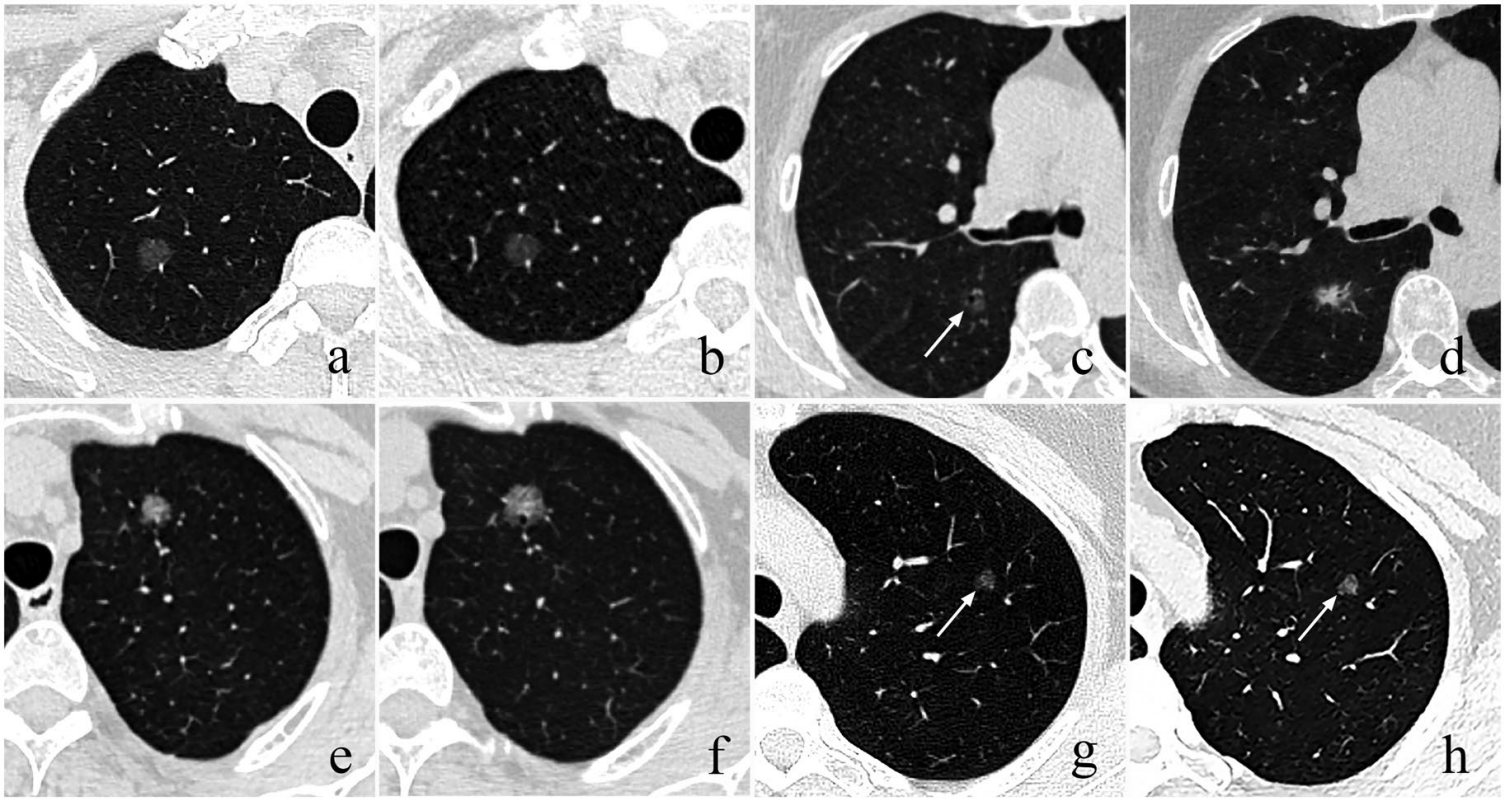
SSNs with different growth patterns. (a, b) A 45-year-old female has a pGGN (diameter: 9.2 mm; volume: 283 mm³; CT value: −690 HU) located in the RUL. On follow-up CT (interval: 36.4 months), it shows no significant growth (diameter: 9.2 mm; volume: 318 mm³; CT value: −684 HU). After operation, it is confirmed as AIS. (c, d) A 75-year-old female has a pGGN (white arrow) (diameter, 8.9 mm; volume, 188 mm³; mass, 82 mg; CT value, −699 HU) located in the RLL. On follow-up CT (interval: 65.6 months), it shows diameter growth of 6.9 mm, volume growth of 1336 mm³, mass growth of 923 mg, and a new 7.2 mm solid component. After operation, it is confirmed as IAC. (e, f) A 58-year-old female has a pGGN (diameter, 9.5 mm; volume,261 mm³; mass, 172 mg; CT value, −451 HU) in the LUL. On follow-up CT (interval: 15.1 months), it shows diameter growth of 2.3 mm, volume growth of 409 mm³, and mass growth of 191 mg, but no significant growth in CT value (−442 HU). After operation, it is confirmed as MIA. (g, h) A 58-year-old female has a pGGN (white arrow) (diameter, 6.7 mm; volume, 104 mm³; CT value, −697 HU) in the LUL. On follow-up CT (interval: 43.4 months), it shows growth in CT value (−637 HU), but no significant growth in diameter (6.7 mm) and volume (107 mm³). After operation, it is confirmed as AIS. SSNs, subsolid nodules; pGGN, pure ground-glass nodule; CT, computed tomography; HU, Hounsfield unit; AIS, adenocarcinoma *in situ*; MIA, minimally invasive adenocarcinoma; IAC, invasive adenocarcinoma; RUL, right upper lobe; LUL, left upper lobe; RLL, right lower lobe.
